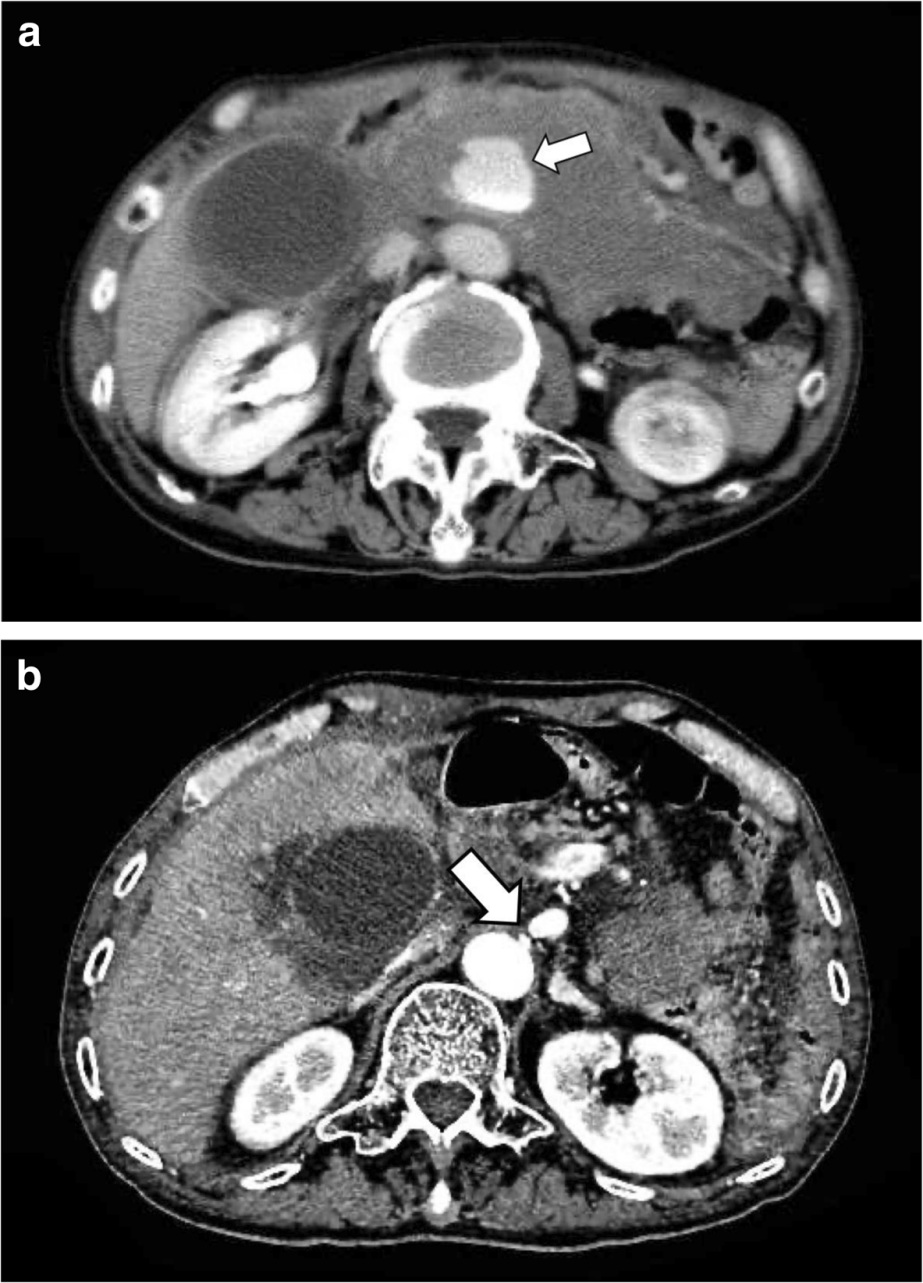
a Computed tomography taken at visiting a physician showed a hematoma, and inferior pancreaticoduodenal artery aneurysm rupture was suspected (shown by arrow). b Computed tomography taken at visiting a physician showed stenosis of the root of the celiac axis (shown by arrow)
